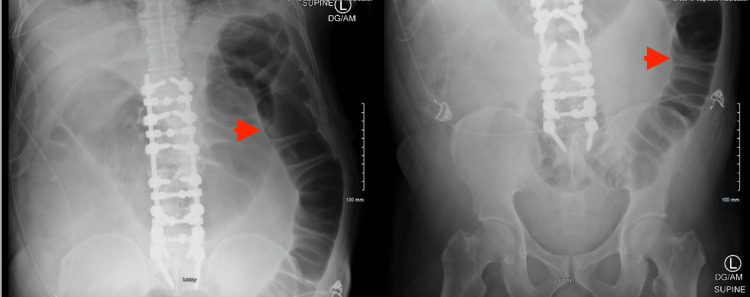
Abdominal X-ray Plain abdominal X-ray showing colonic dilation. It also showed the hardware of the spinal fusion surgery (red arrowheads)
